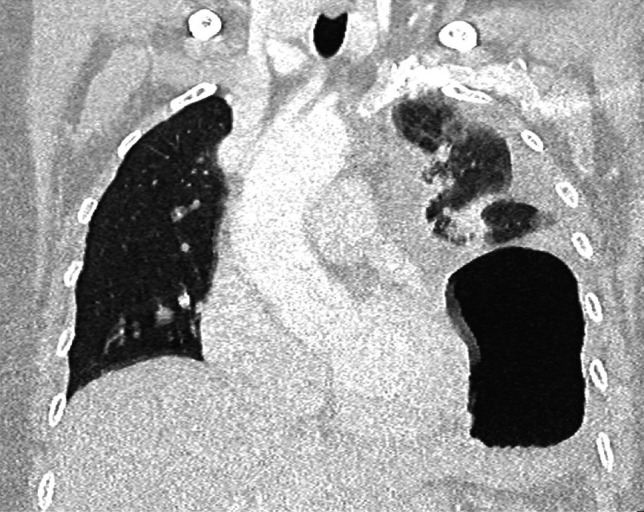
Computed tomography scan of chest demonstrating left diaphragmatic hernia
